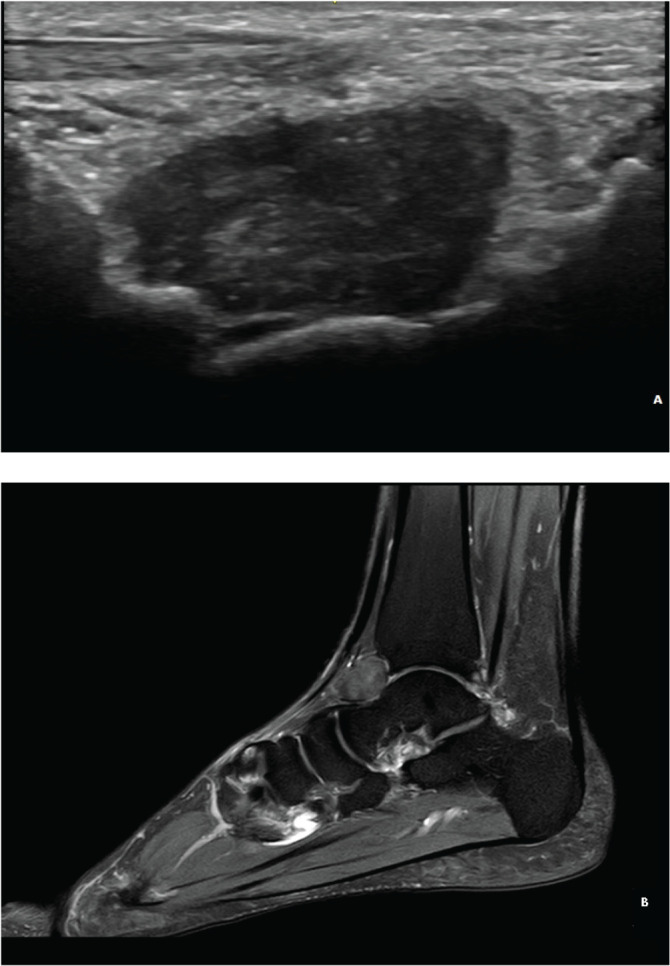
Ultrasound Sonography of the RT ankle-joint showing heterogeneous lesion with irregular margin to the outer front portion of the ankle (A). Magnetic Resonance Imaging scan (T1-W) demonstrating a solid heterogeneous lesion with dimensions 1.6x2.5x2.8cm (B).
